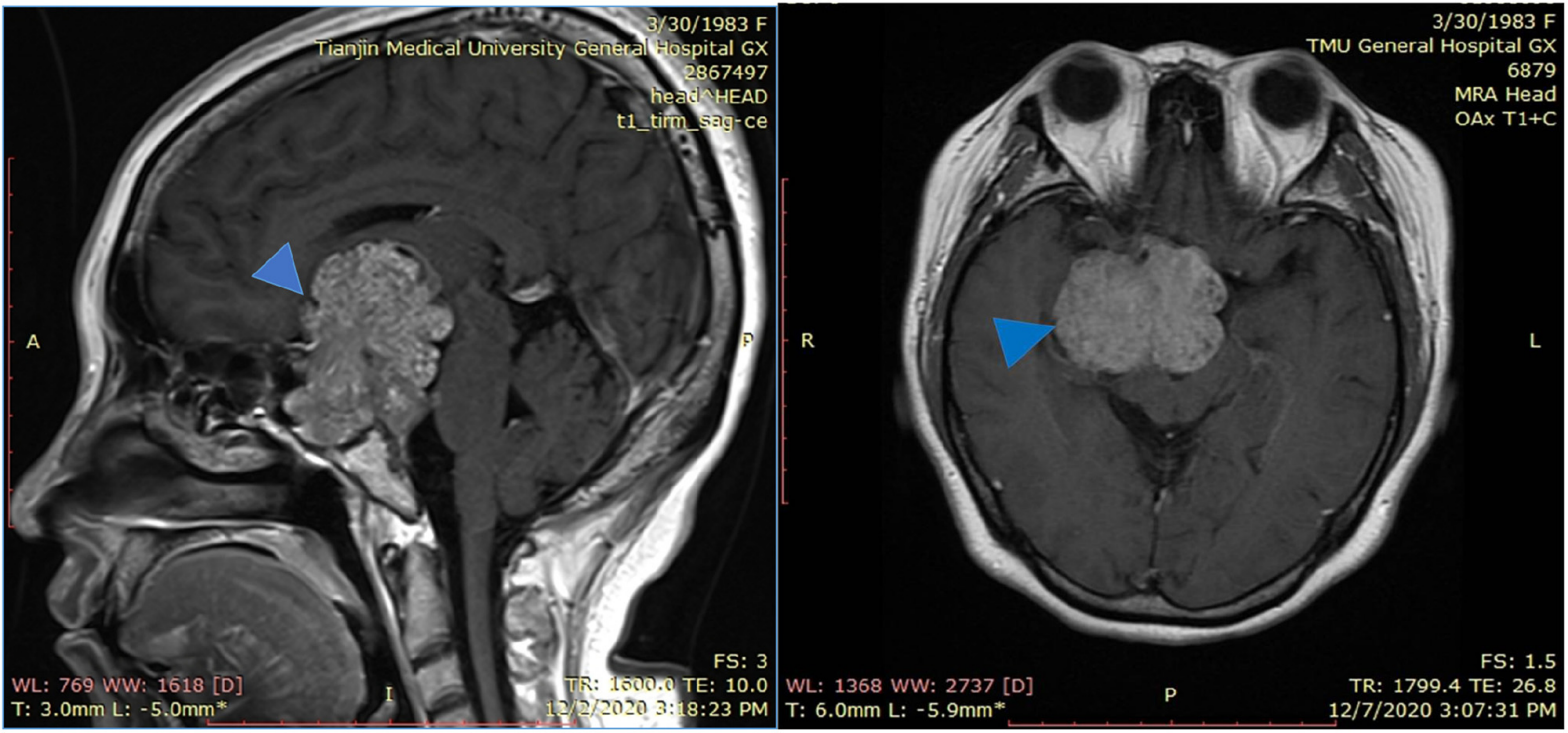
Enhancement of the pituitary gland determined via MRI. The tumor was located in the sella turcica and suprasellar region (arrows).
